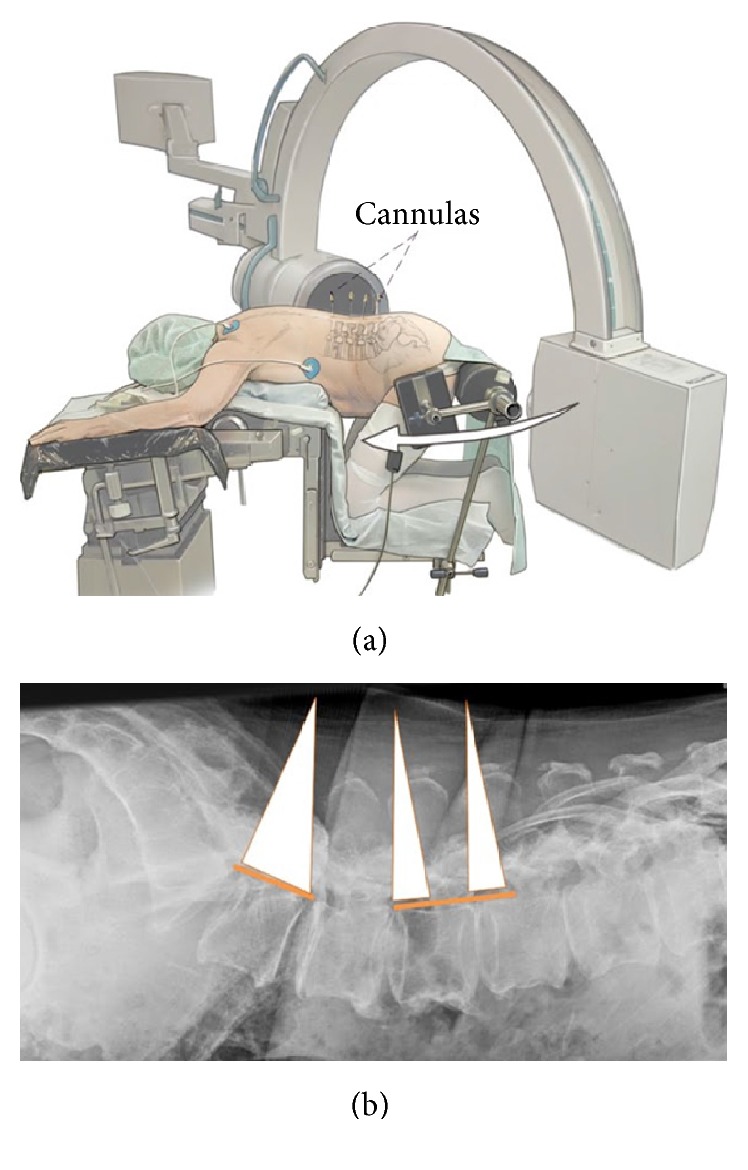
(a) From [8] with permission. Localization of the levels to be approached. Cannulas are inserted to mark the levels under fluoroscopic control. (b) From [8] with permission. Lateral X-ray with graphic marking of the approach corridors to the levels L3-4-5-S1. Due to the lordotic angle of the lumbosacral junction, the levels L4-5-1 can be approached through one small incision while the other two levels are approached through separate skin incisions (“Giant Slalom”).
